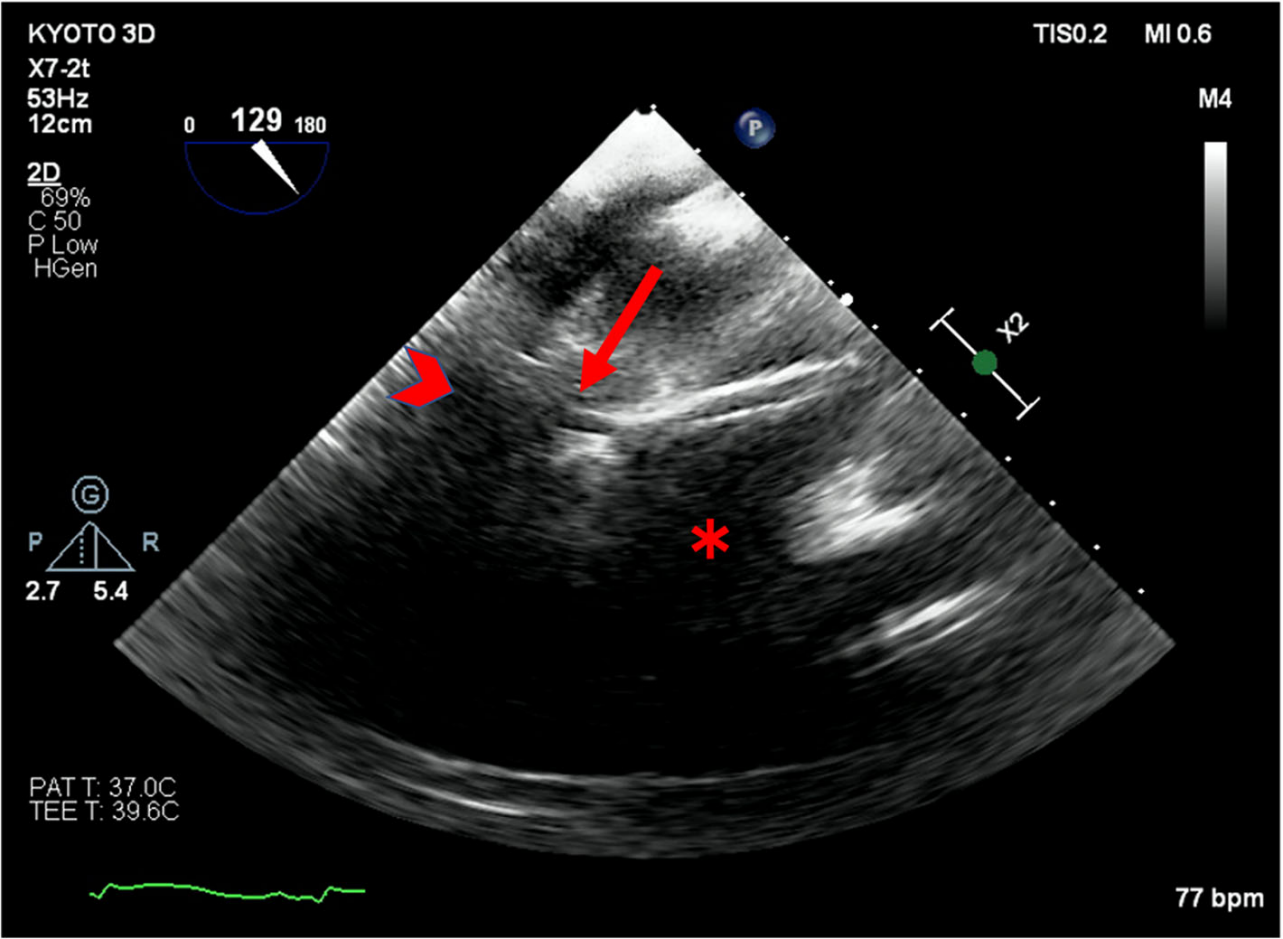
A straying tip of the IMPELLA® in the left ventricle apical wall detected by transgastric long-axis view by transesophageal echocardiography. Note the massive pericardial effusion (arrow head) and the two highly echogenic parallel lines indicating the tip of the IMPELLA® and the thinning left ventricular apical wall (small arrow). Left ventricle anteroseptal wall was not depicted probably because of acoustic shadow of the IMPELLA® (asterisk)
